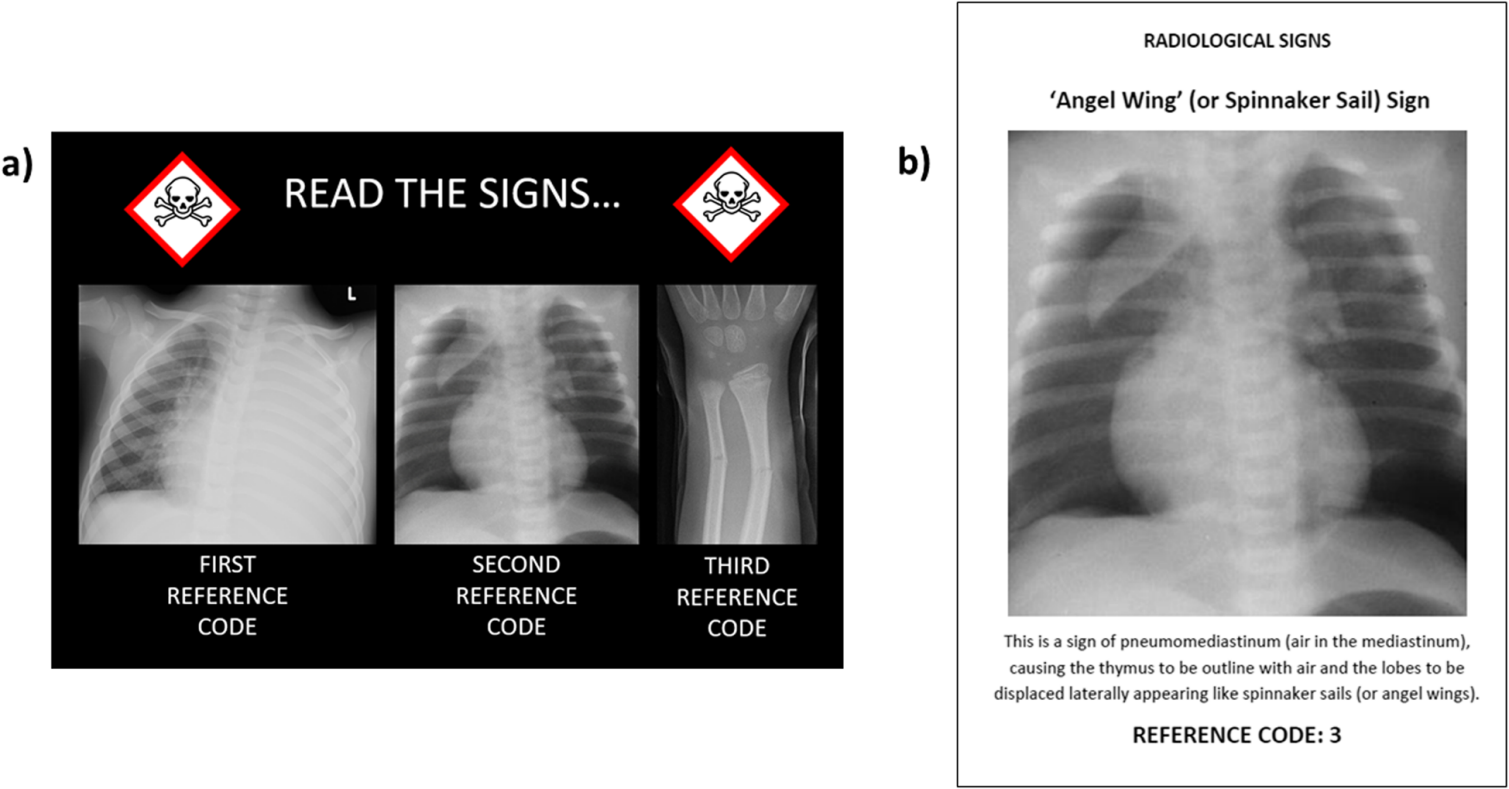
‘Read the signs’: an example of one of the puzzles in the escape room. a A poster detailing three different paediatric radiographs with abnormalities is placed on one of the walls of the room. The students need to find a hidden folder of ‘radiology signs’ somewhere in the room containing 20 different imaging signs and match the images on the poster to that in the folder to solve the three number combination code. b An example of one of the pages in the clear plastic presentation folder matching the second image on the poster. In this example, the second number for the combination code would be ‘3’
